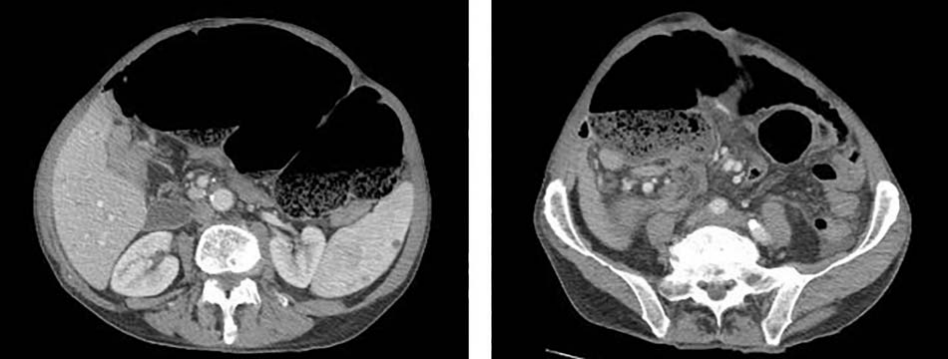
CT of the abdomen and pelvis demonstrating coffee-bean shaped cecum in left upper quadrant dilated to 12 cm, consistent with cecal volvulus.
